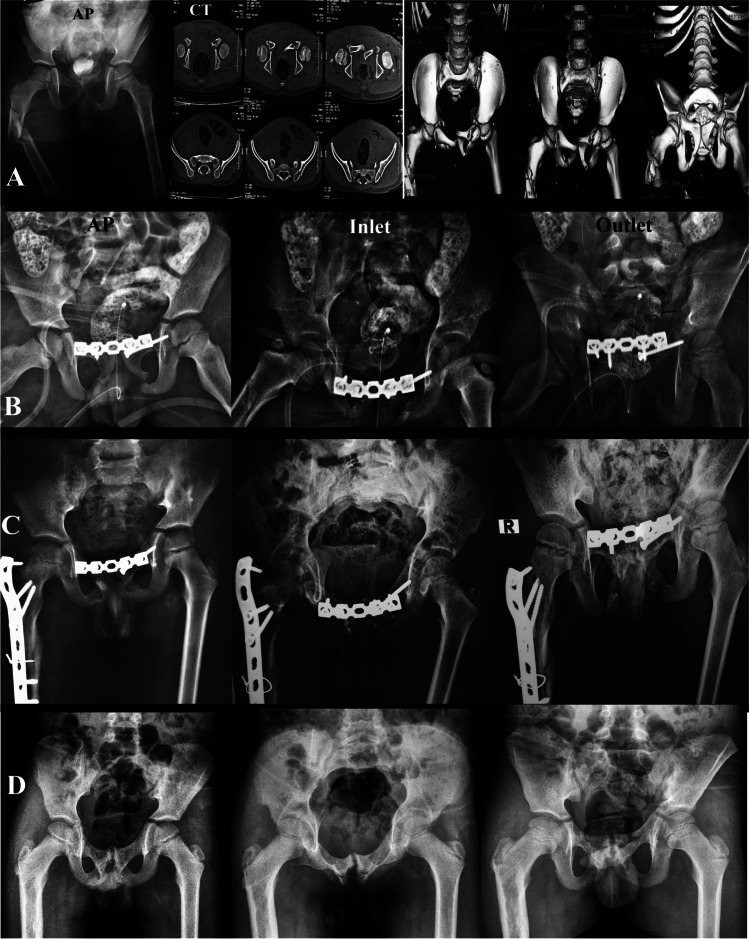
Male child 8 years old presented after a motor car accident with fracture pelvis type B2.2 (Lt crescent fracture, anterior symphyseal disruption, Lt pubic rami fracture) treated with a symphyseal plate. Associated injuries (fracture Rt femur, urethral injury) ORIF of the femoral fracture was performed in another session. A Preoperative imaging studies (plain AP pelvis radiograph and a CT scan). B Immediate post pelvic fracture fixation. C After 15 months of follow-up. D After 29 months of follow-up (hardware was removed)
